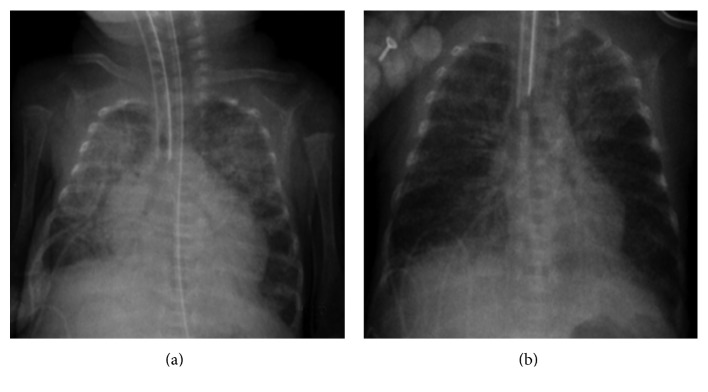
Acute (a) and convalescent (b) chest radiographs demonstrating cardiomegaly at the time of the clinical deterioration, with near complete resolution five days later.
